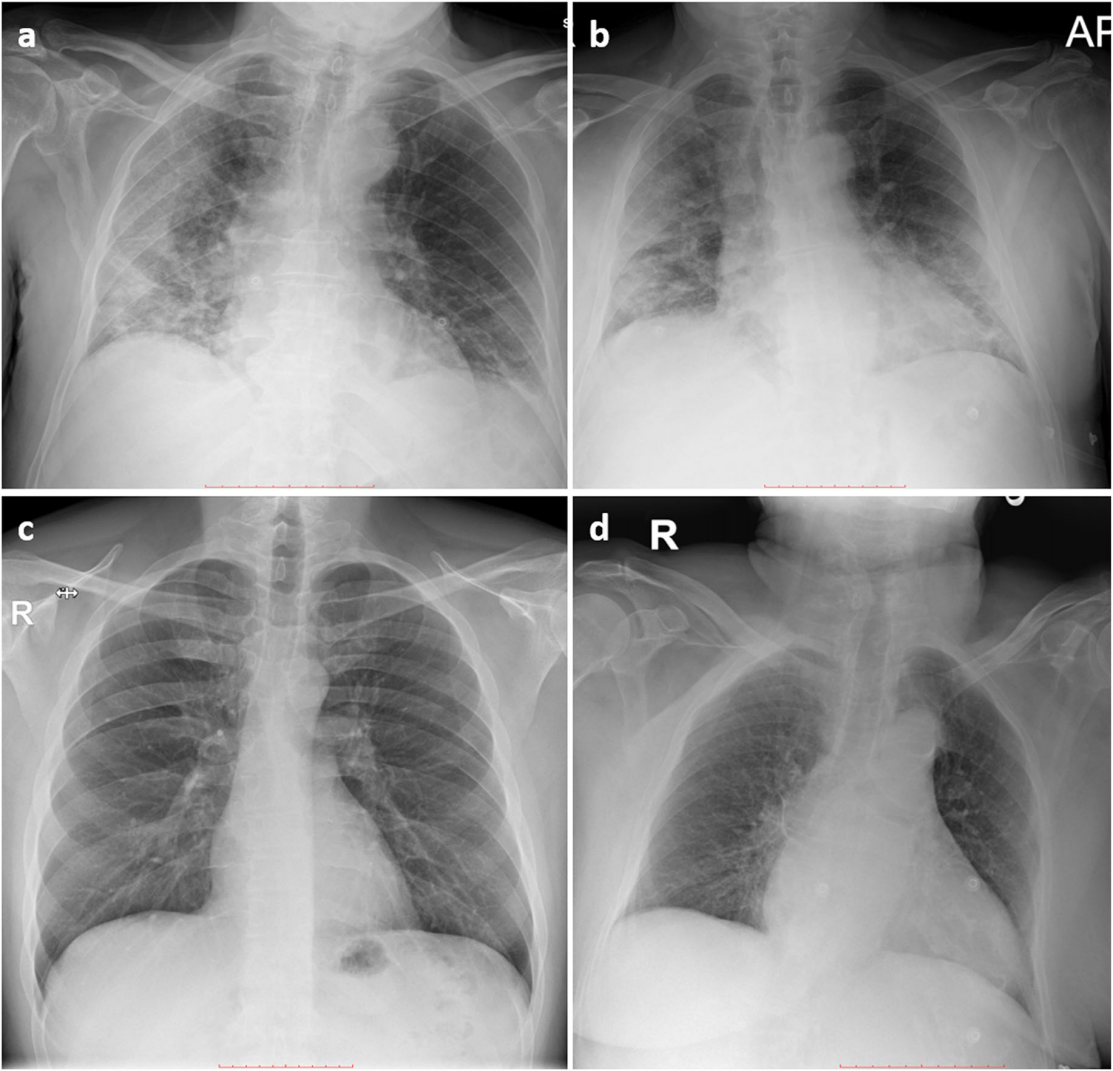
Sample chest x-ray examinations from patients included in the study sample. Posteroanterior projection in a COVID-19-positive 75-year-old male patient (a), bedside anteroposterior projection in a COVID-19-positive male patient (b), posteroanterior projection in a negative 42-year-old male patient (c) and bedside anteroposterior projection in a negative 88-year-old female patient (d)
